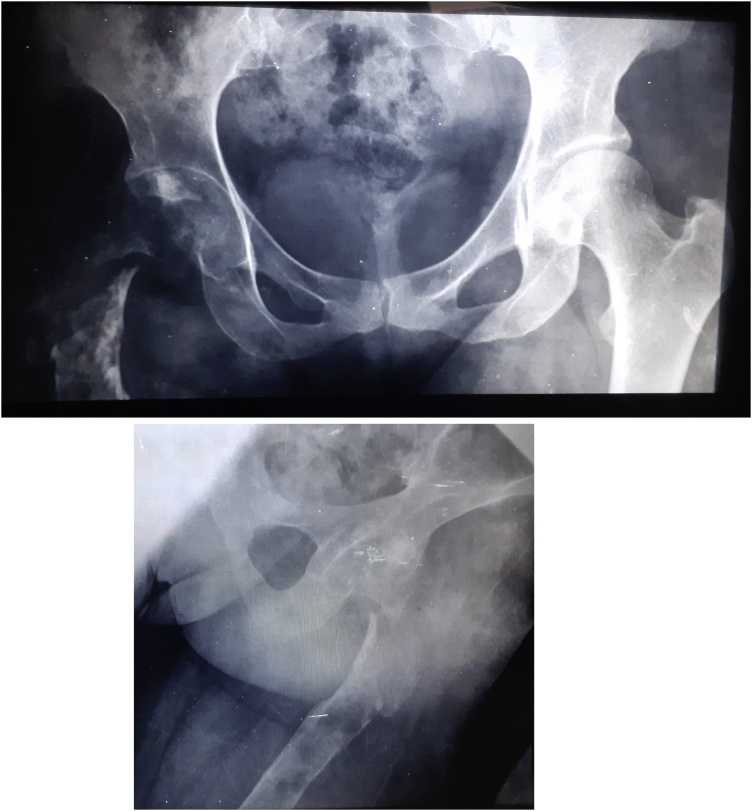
X-ray images after removing the osteosynthesis material.
